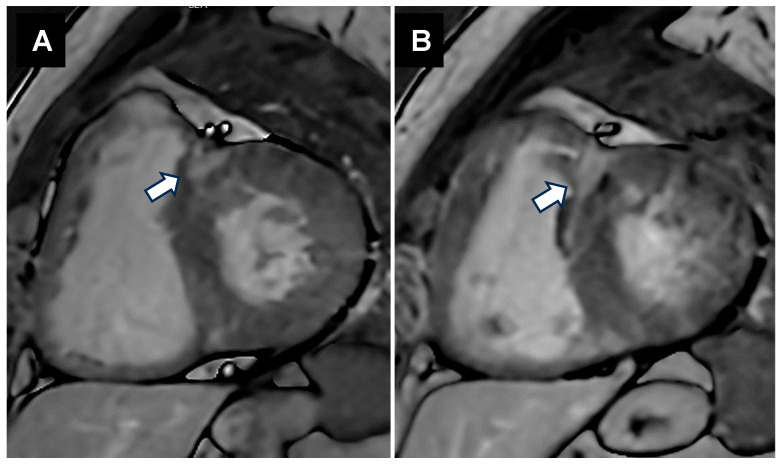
Cardiovascular magnetic resonance images of patients with rheumatic mitral stenosis during diastole (A) and systole (B). White arrows indicate regions of late gadolinium enhancement (LGE).
